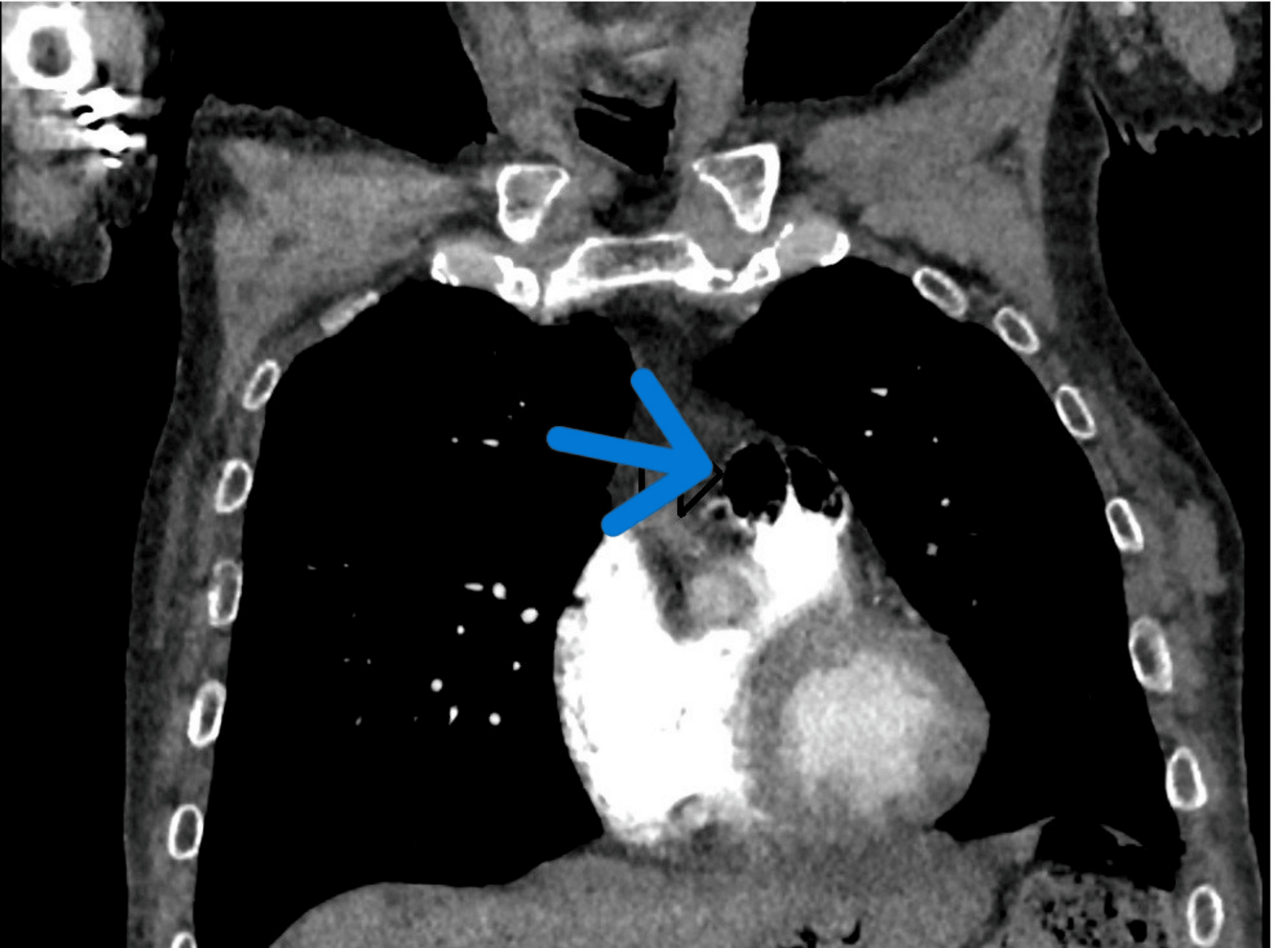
Sagittal view of computed tomography (CT) scan of the chest, showing a small to moderate volume of air embolism (indicated by the arrow) within the main pulmonary artery and right ventricle.
